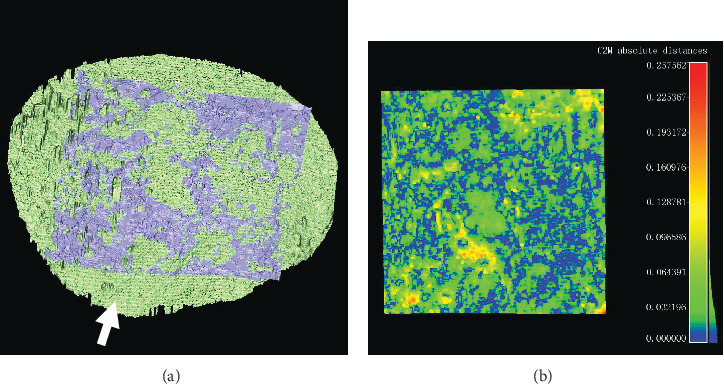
(a) Registration of two tidemark surfaces obtained from the ultrasound (gray) and micro-CT (green) imaging. The white arrow shows the direction of the inserted metal pin. (b) Clouds/mesh distance mapping for ultrasound obtained tidemark surface to that from micro-CT after registration. Shown on the right is the color bar used for distance mapping in unit of mm. An averaged distance discrepancy of 26.8 ± 22.9 *μ*m (mean ± SD) was obtained in this case.
